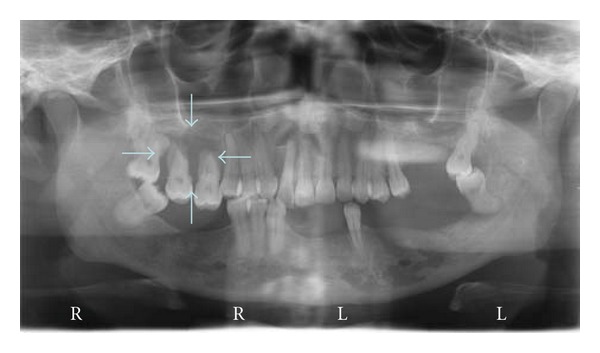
Case 4. Panoramic radiograph showing lytic area with floating teeth. Maxilla.
